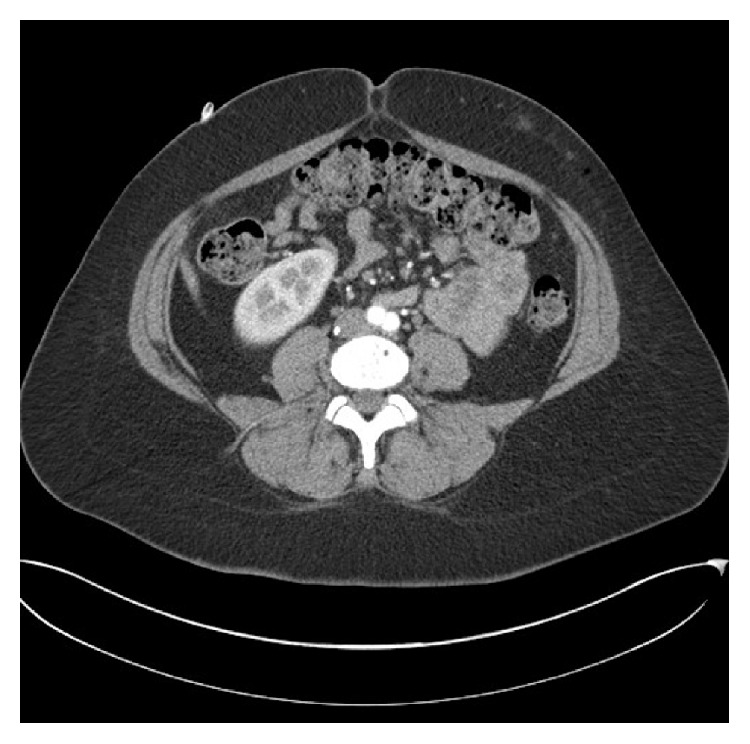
Preoperative CT angiogram of Patient 2 demonstrating subcutaneous opacities, representing subcutaneous bleeding from heparin injection over the left abdomen. Note the close proximity to DIEP perforators.
